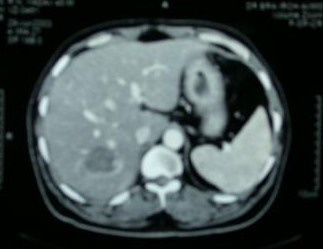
Post radiofrequency ablation CT scan image showing complete resolution of hepatic metastasis.
